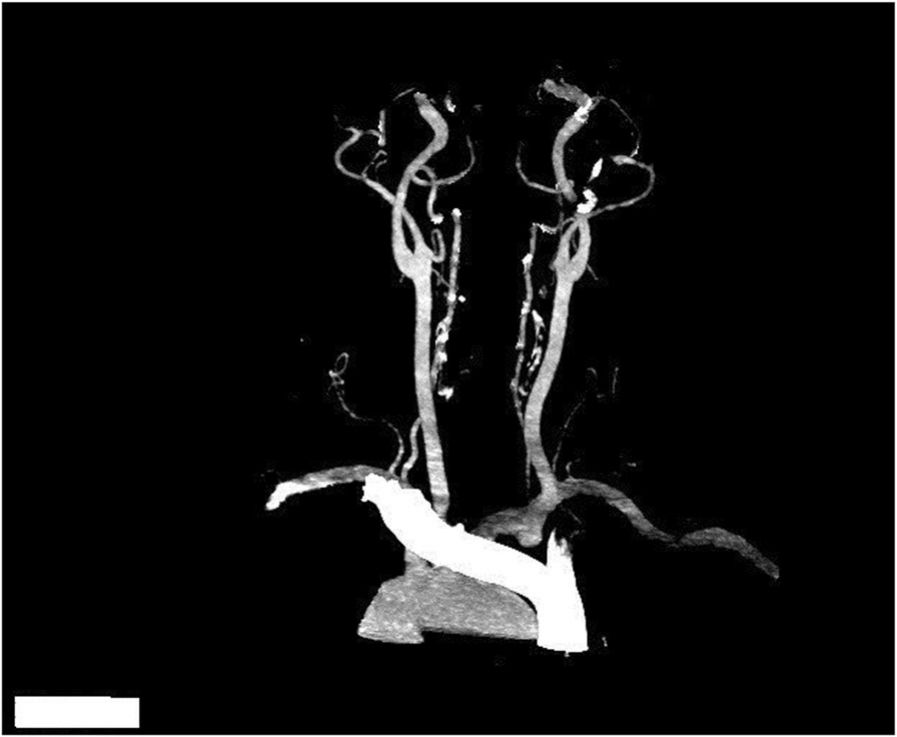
CT angiography of neck. CT angiography of the neck shows that there is no malformation of neck vessels
